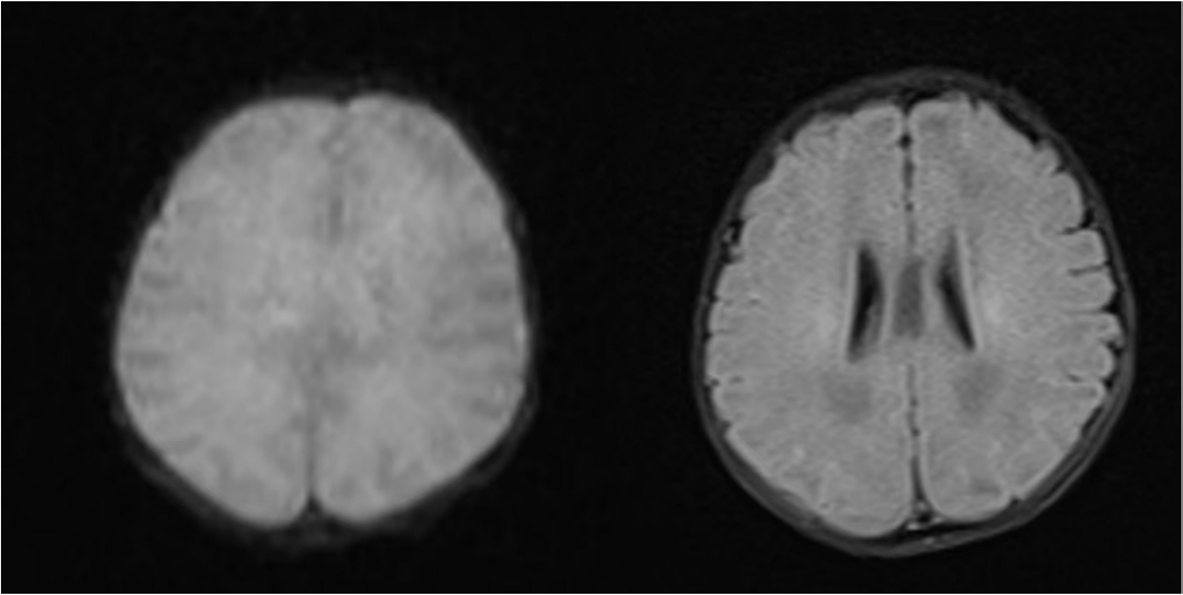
Brain MRI plain scan and diffusion-weighted imaging of the patient
